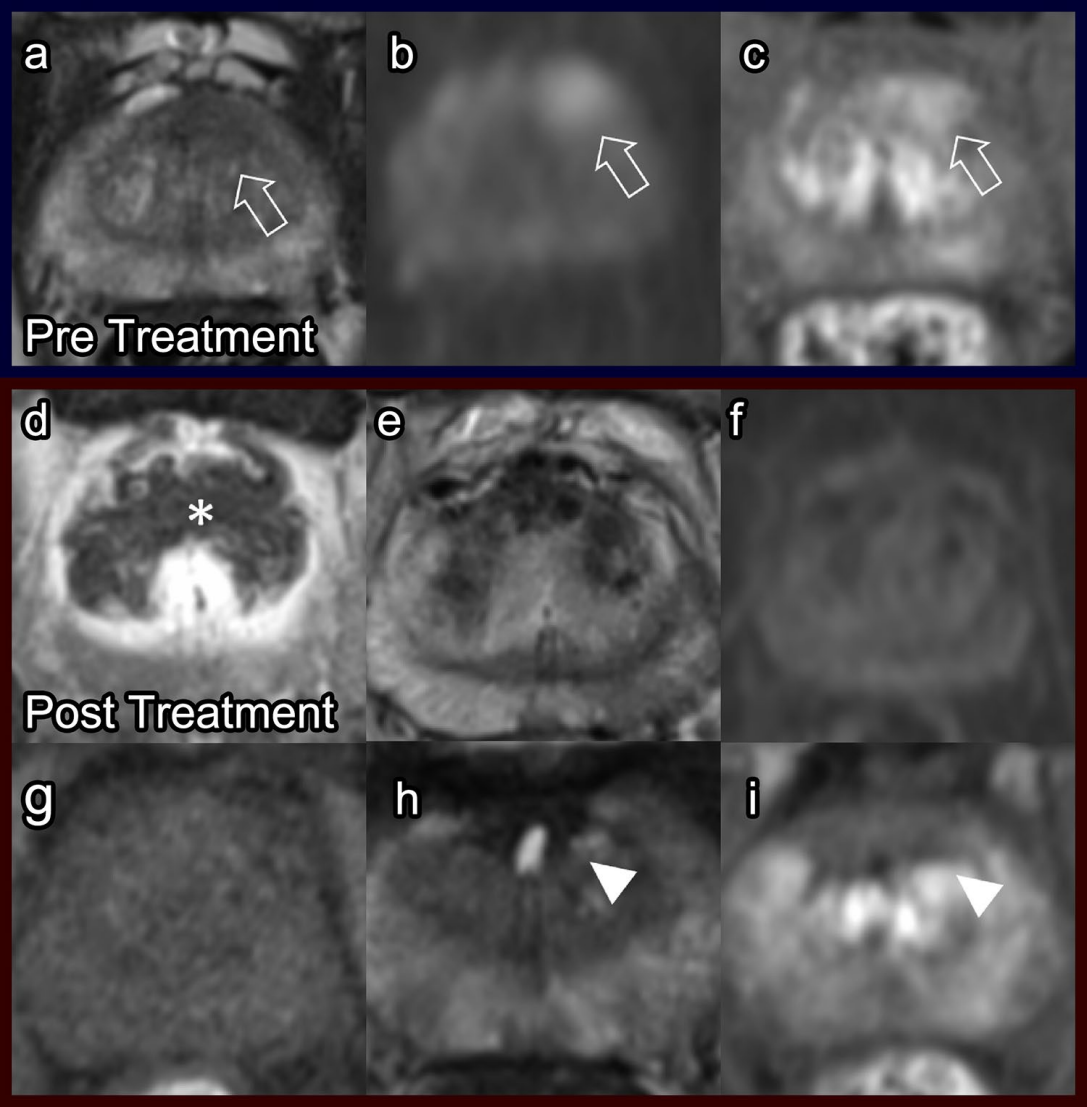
Irreversible electroporation. Pre-treatment prostate MRI: Axial T2 (a), DWI (b) and post-contrast T1 (c) demonstrate an anterior left mid-gland lesion (arrows). Biopsied as Gleason score 3 + 4 prostate cancer. Post-IRE prostate MRI at 7 days: DCE (d), T2 (e), DWI (f), and pre-contrast T1 (g) show a post-procedure necrotic area (asterisk) and heterogeneous decreased T2 signal. Post-IRE prostate MRI at 3 years: T2 (h) and DCE (i) indicate residual fibrosis (arrowheads), with no signs of recurrence at the treatment site
